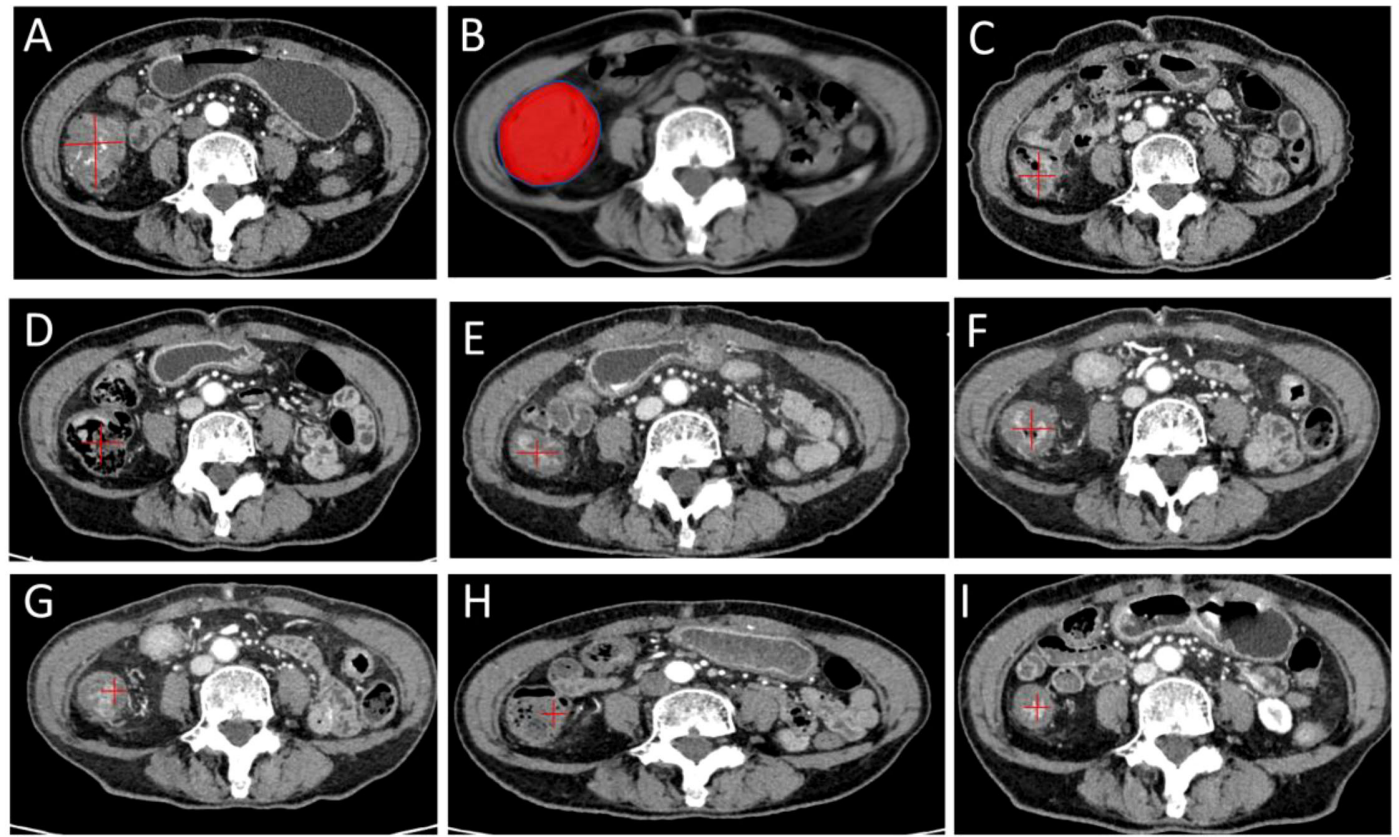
Serial CT imaging (1mm) of an ascending colon cancer lesion during oART: Baseline contrast-enhanced CT before treatment (A); Online adaptive radiotherapy (ART) dose distribution, showing the 100% prescription isodose (red) providing conformal coverage of the planning target volume (B); Follow-up CT scans obtained at various time points (26, 75, 120, 200, 230, 263 and 330 days post-radiotherapy) demonstrating continuous tumor regression (C–I).
